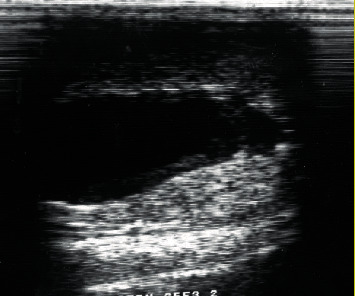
Ultrasound image of intratesticular spermatocele in a Dama gazelle.
